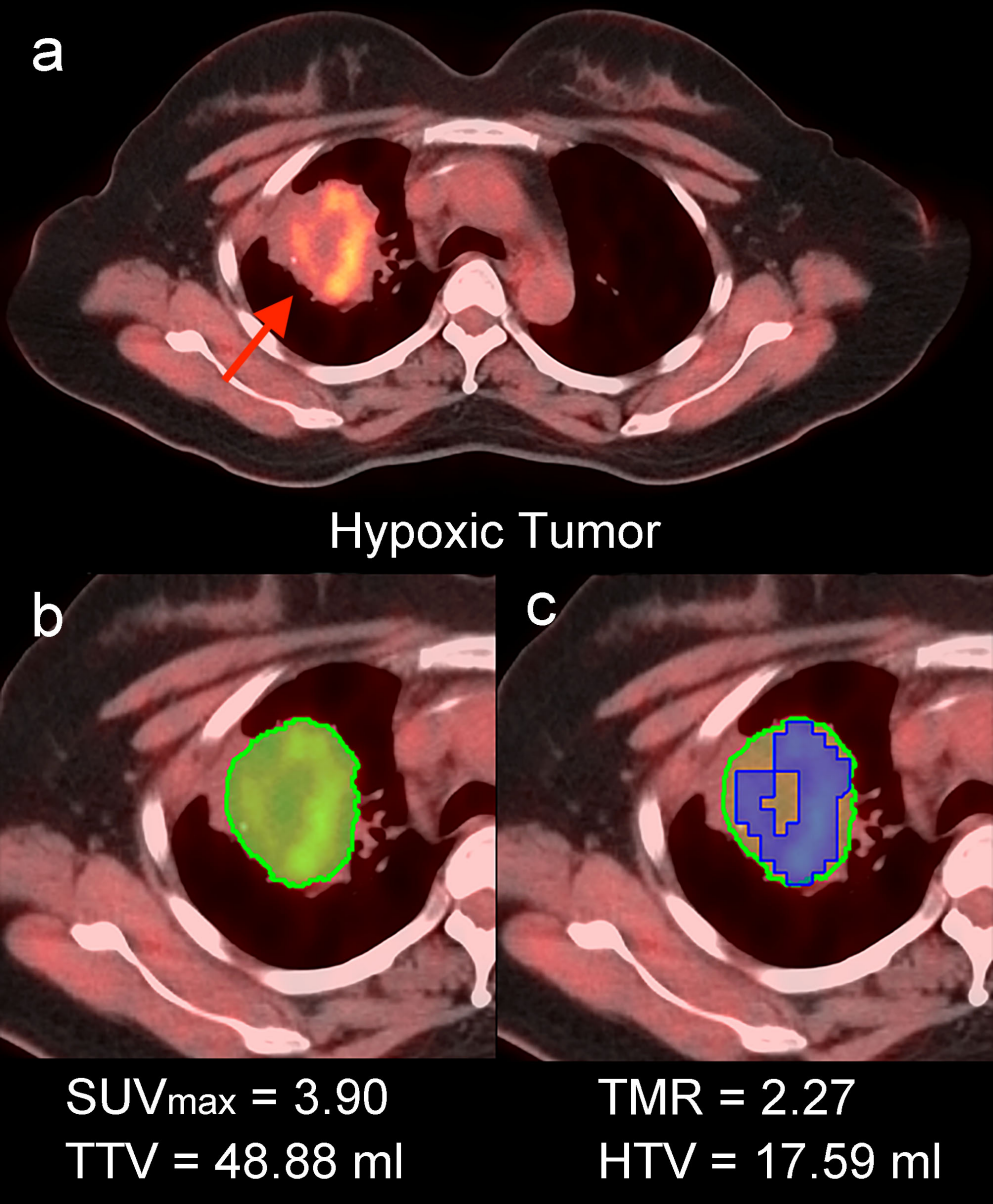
^18^F-FMISO PET/CT image analysis on the MIM software. Hypoxic tumor was defined (red arrow) by obvious ^18^F-FMISO uptake (a) and semi-automatedly segmented (green area) with TTV and SUVmax calculated (b). The TMR was subsequently calculated (c) and the HTV was also defined (blue area).
